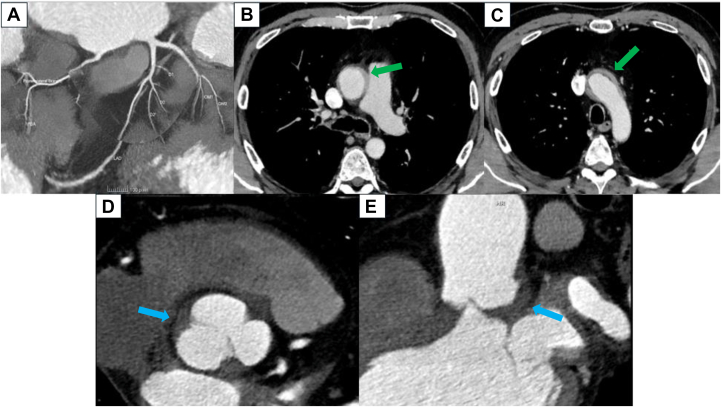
CT Scan (A) No coronary artery disease. (B and C, green arrows) Crescent-shaped mural thickening of approximately 7 mm along the aortic wall, extending from the root to the arch. (D and E, blue arrow) At the level of the annulus, the mural thickening extended into the aorto-mitral junction. CT = computed tomography.
